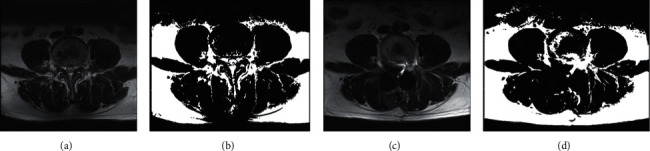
A 45-year-old male patient diagnosed with lumbar spinal stenosis. (a) represents L4-L5 vertebral MRI, while (b) is the same image obtained after processing by the ImageJ software. (c) represents L4-L5 vertebral MRI after CBT surgery, while (d) is the same image obtained after processing by the ImageJ software. The area enclosed by the yellow line after image thresholding in the ImageJ software is the ES portion of the paravertebral muscle. ES area and FI obtained by calculation using the ImageJ software.
